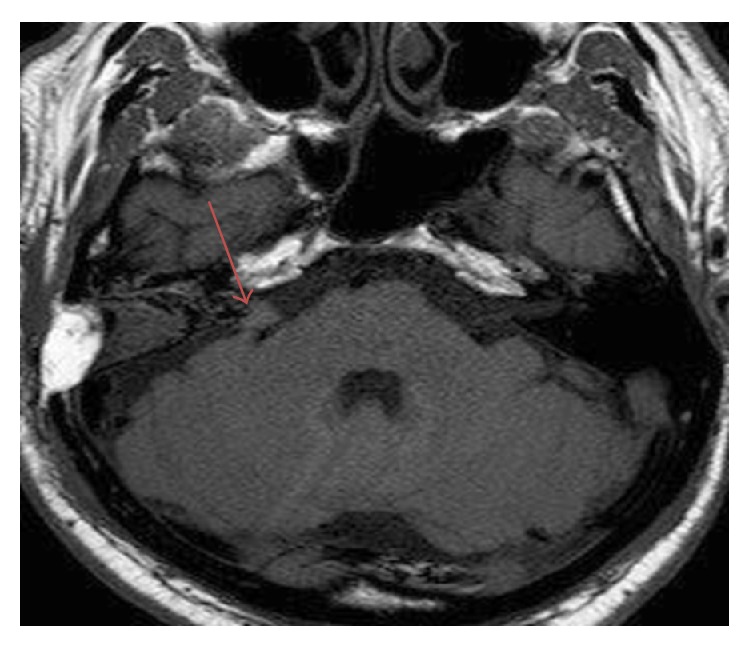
MRI-scan (axial section) demonstrating a contrast enhancing lesion in the right internal auditory canal.
